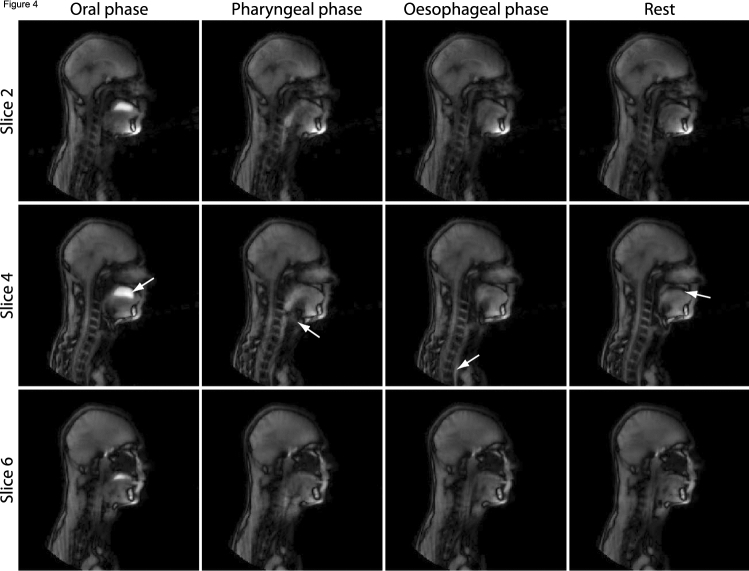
Overview of real-time 3D MRI of swallowing four frames (at equal distance in time) that represent four swallowing phases. Three of the total of seven slices are shown. The contrast agent (pineapple juice) is displayed as a hyperintense fluid in the oral phase (arrow in the first column). Laryngeal elevation and closure by the epiglottis can be appreciated in the pharyngeal phase (arrow in second column). In the oesophageal phase, the contrast agent is located in the oesophagus (arrow third column). Finally, in rest, tongue relaxes and creates space between the palate and itself (arrow last column)
